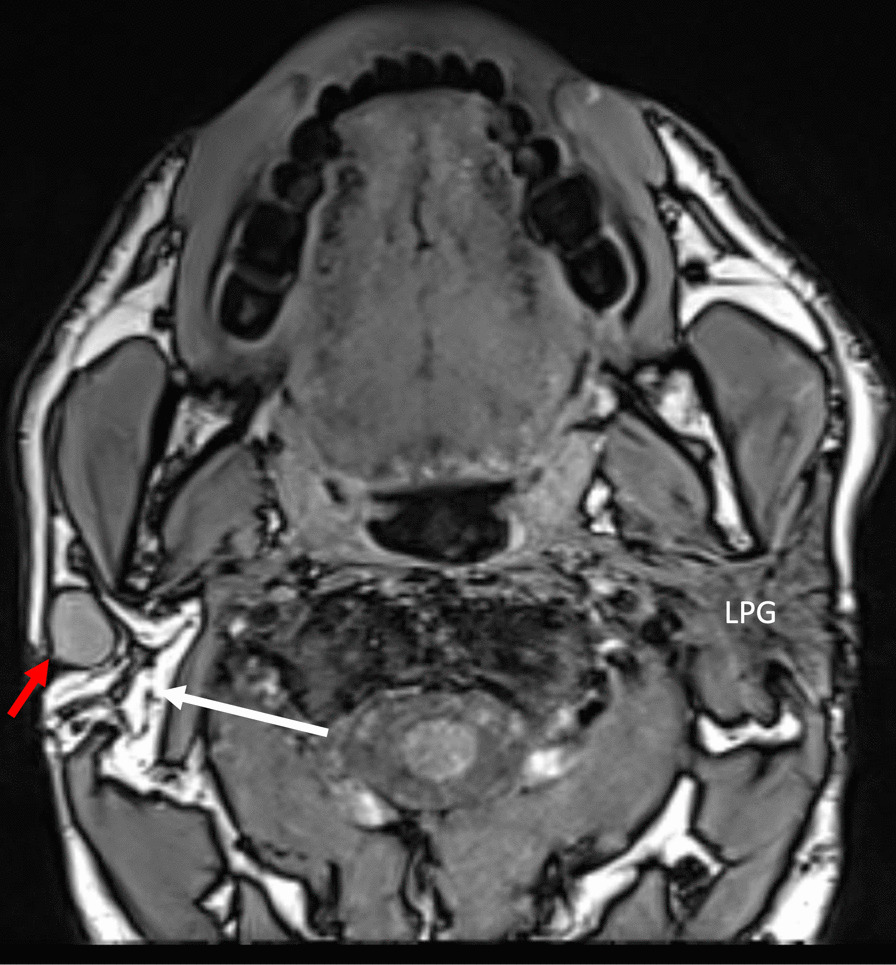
Magnetic resonance imaging of the neck with gadolinium (T1 sequence) revealed a normal left parotid gland (LPG). In the right parotid space, no parotid gland was noted. Instead, a rather homogeneous well-defined mass (indicated by the small red arrow) was surrounded by fatty tissue (indicated by the longer white arrow)
